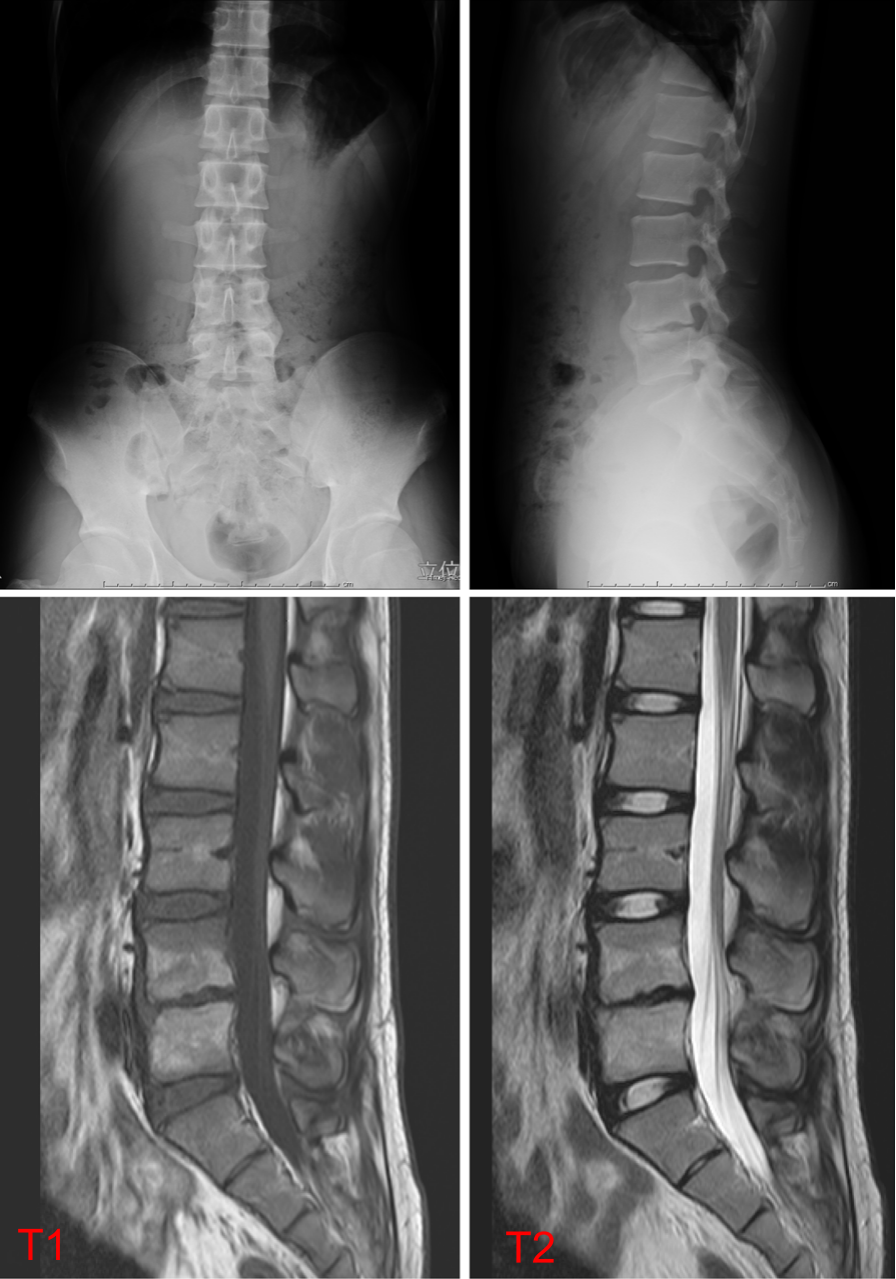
The radiograph and MRI at the point of 6 months follow-up after discharge revealed improvement of vertebral bodies alignment, and no exacerbation of abscess formation or bone destruction
